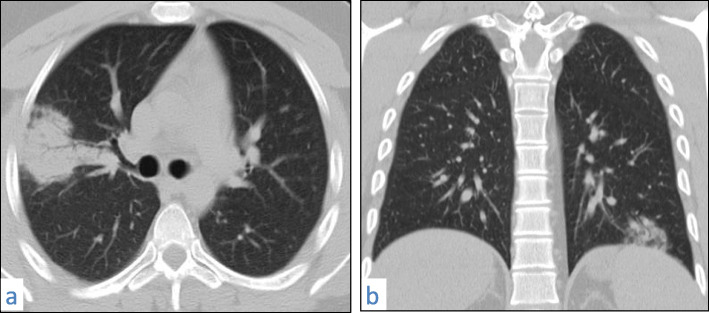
A 31-year-old male patient with COVID-19, CT chest axial image (a) shows right upper lobe predominantly subpleural consolidation with air bronchogram, coronal image (b) shows left lower lobe subpleural consolidation with traction bronchiectasis and a tiny lucency “vacuolar sign”
